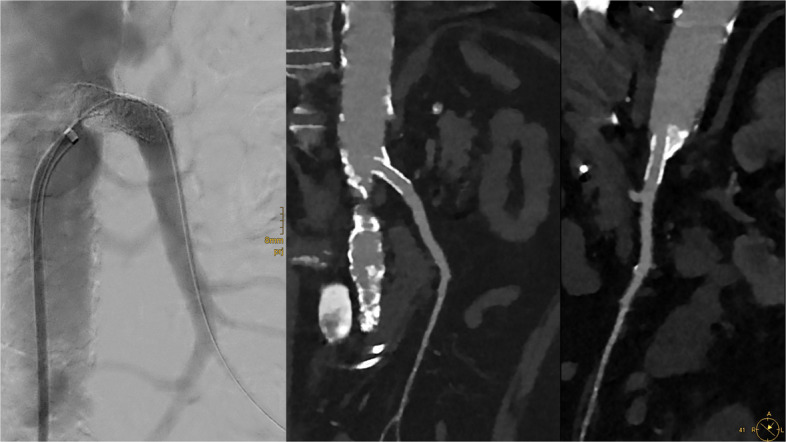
Post-treatment angiography following IVL and implantation of an 8.0 × 24 mm Formula balloon-expandable stent demonstrates successful revascularization of the SMA. Multiplanar reconstruction of the follow-up CTA after Ivor Lewis esophagectomy (14 days post stenting) confirms sustained vessel patency without evidence of restenosis or perioperative vascular compromise. Residual stenosis was 22%
